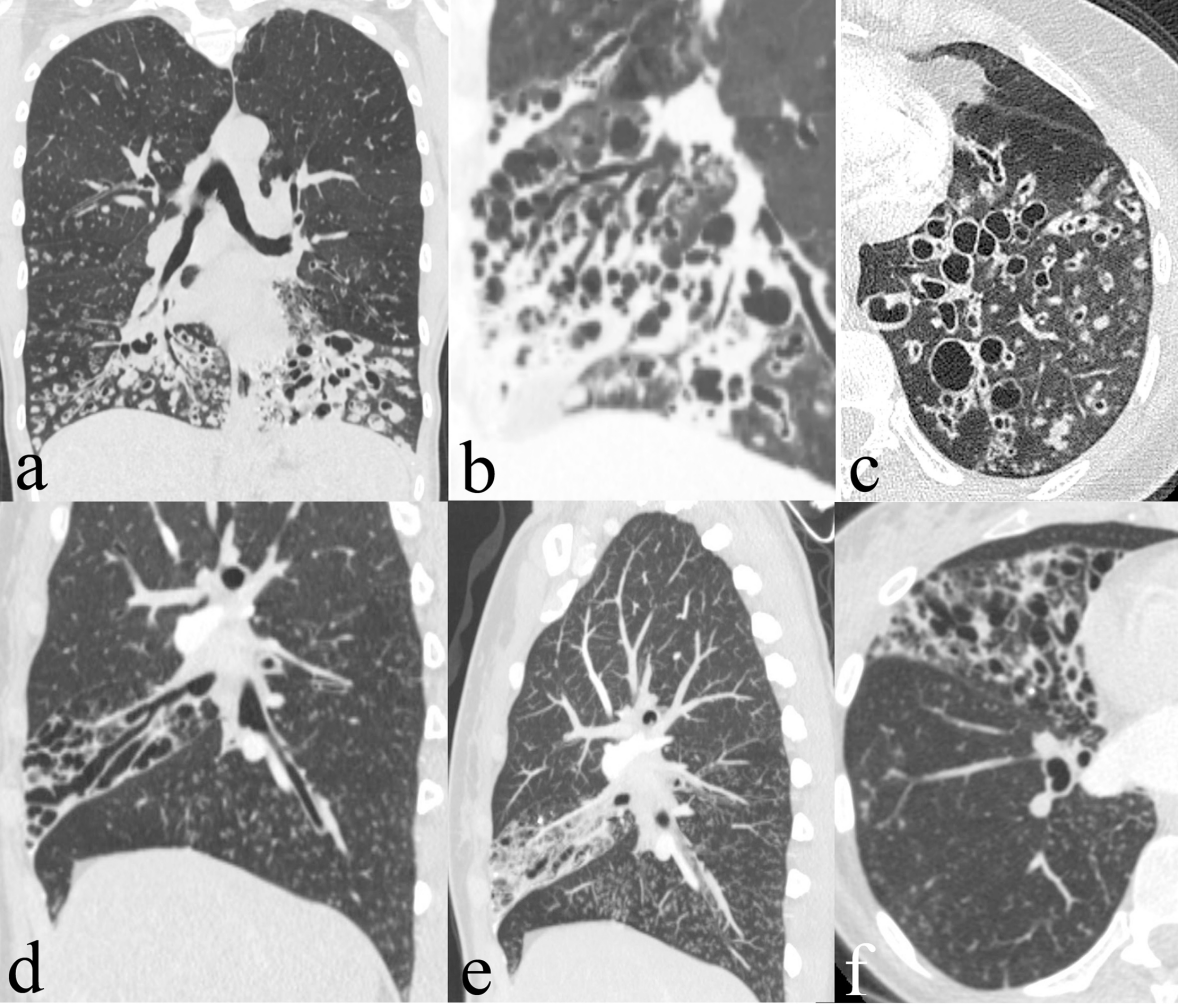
CT in patients with PCD. CT of two patients with definite PCD. Patient 1 (a-c) is a 34 year old female patient with PCD. CT shows cystic bronchiectasis especially in the lower lobes, severe mucous-plugging and tree in bud pattern. Patient 2 (d-f) is a 33 year old female patient. CT shows varicose bronchiectasis solely in the middle lobe, mucous-plugging and associated tree in bud pattern in the right lower lobe.
